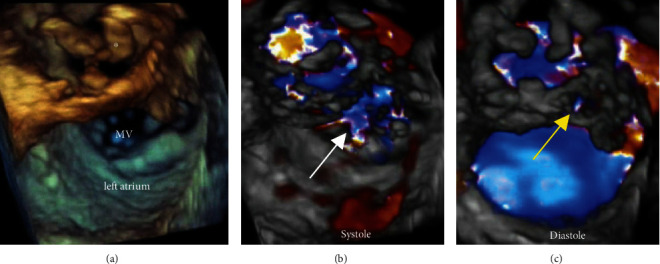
Three-dimensional transesophageal echocardiography. (a) Reference three-dimensional echocardiography showing thickened aortic valve leaflets (∗). (b, c) Three-dimensional color Doppler images suggesting continuous flow across the aorto-mitral continuity in both systole ((b) color denoted by a white arrow is multifactorial from mitral regurgitation and fistula flow) and diastole ((c) yellow arrow) supporting the diagnosis of aorto-atrial fistula.
